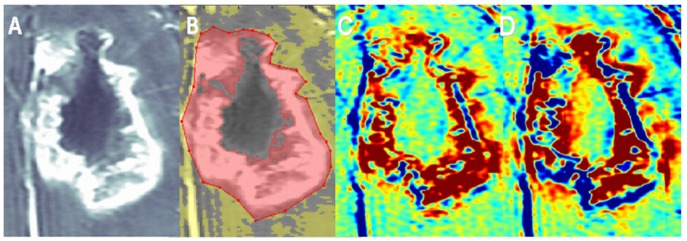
Enhancing lesion volume. Contrast-enhanced T1-weighted MRI without (A) and with (B) a mask selecting the enhancing portion of a GBM tumor (patient #4) are shown. The enhancing lesion volume was calculated from the pixels marked pink in (B). Enhancement subtraction maps calculated at 15 min (C) and 75 min (D) demonstrate the contributions of the red/non-tumor and blue/tumor contributions to the enhancing lesion volume.
